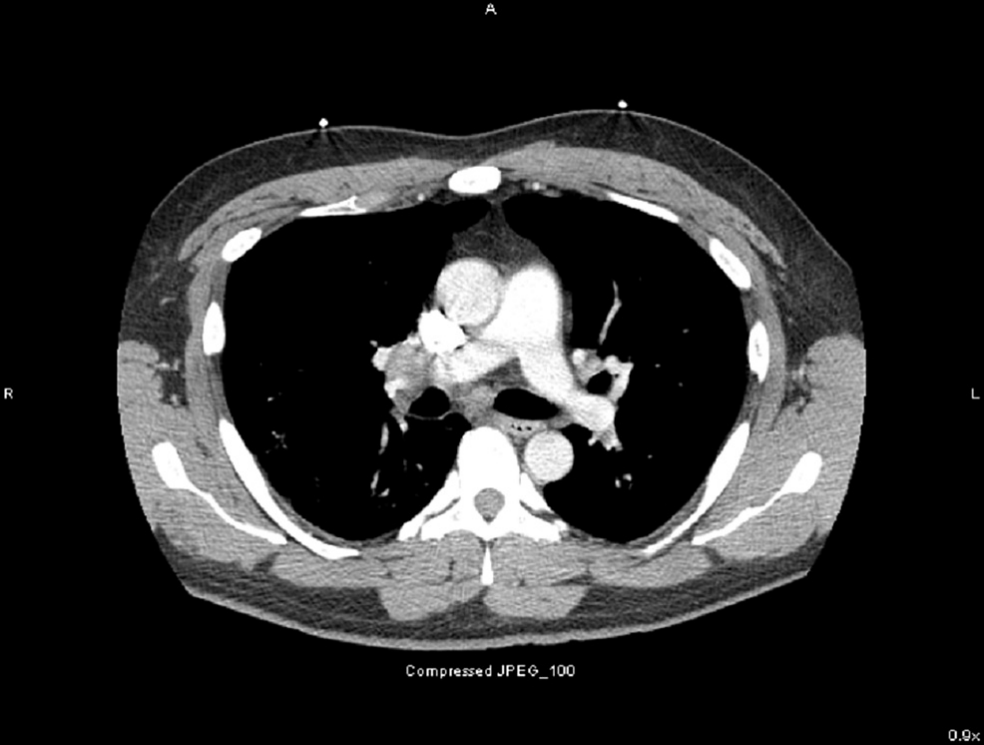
CT of the chest with subcarinal lymph node enlargement.
